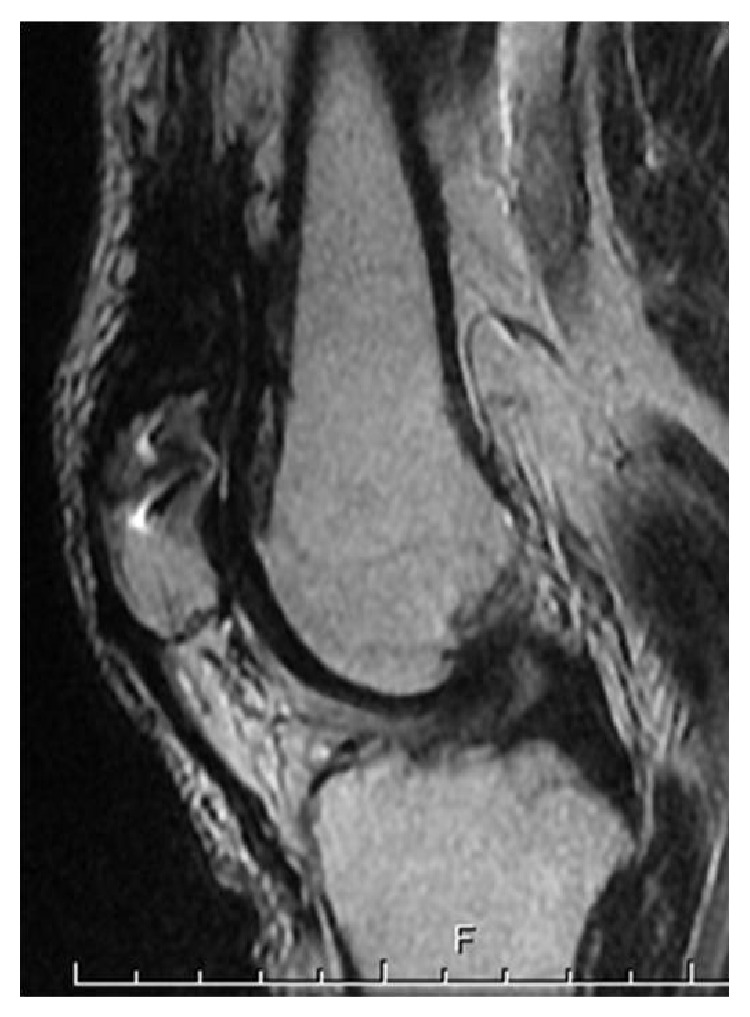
Sagittal postoperative MRI proton density-weighted image. Continuity of the tendon was maintained.
